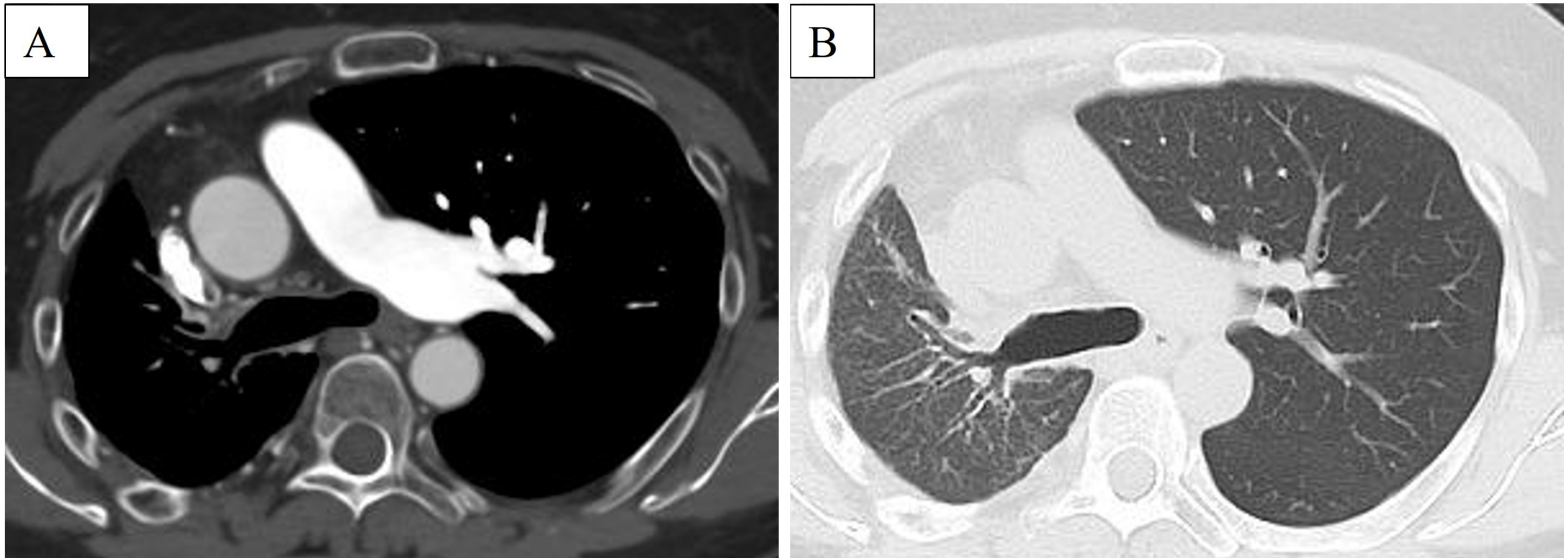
Enhanced chest CT. Chest CT shows absence of the right pulmonary artery and right lung volume loss (A: mediastinal window; B: lung window). CT: computed tomography.
